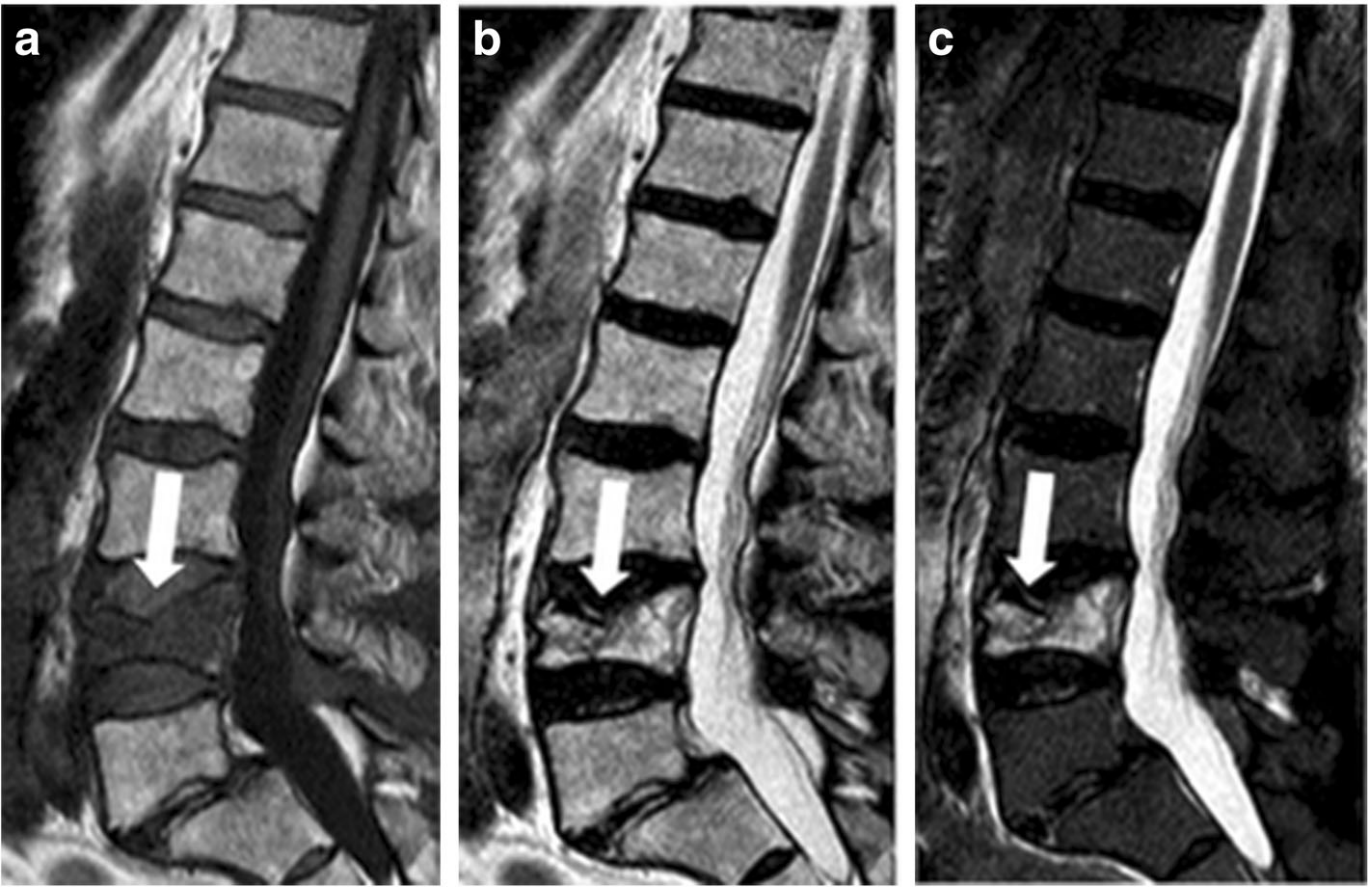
A 72-year old male/female patient with lumbar vertebral fracture treated with image-guided elastoplasty. MRI before treatment: sagittal T1-weighted (a), proton density-weighted (b), and T2-weighted (c) images. A lumbar vertebral fracture in L4 is clearly visualised as decreased signal of the fractured vertebral body in (a) (arrow, a) and increased signal in fractured vertebral body in (b) and (c) (arrow)
